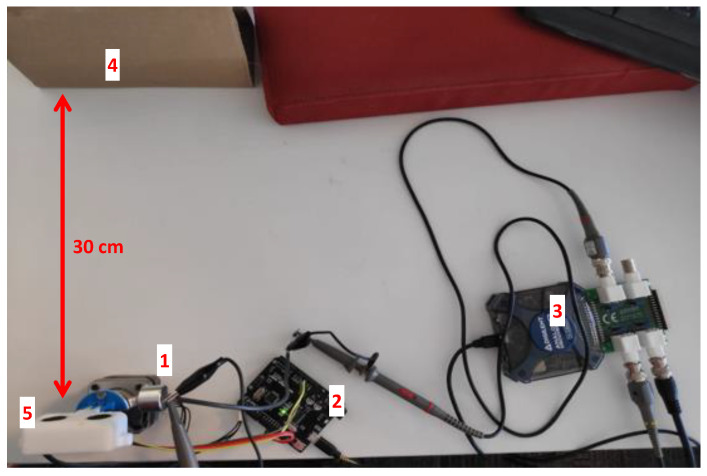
Experimental setup: 1—ultrasonic transmitter; 2—sensor controller; 3—function generator; 4—object; 5—sensor.
